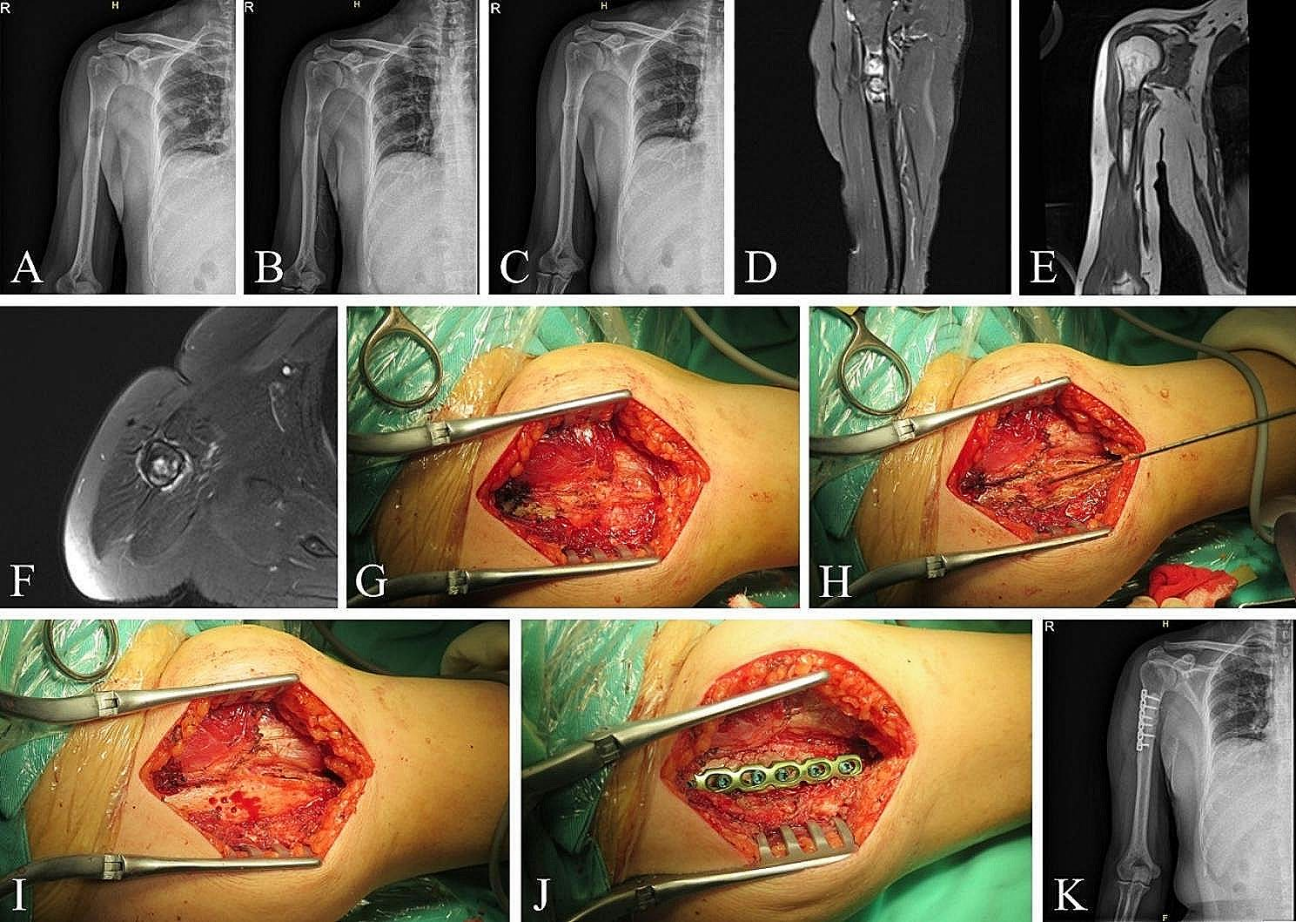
Right humeral pathological fracture in the upper segment. X-rays taken 5 months (A), 4 months (B), and 1 week (C) preoperatively show local thinning of the bone cortex and discontinuity of part of the bone cortex. (D-F) MRI taken 3 months preoperatively shows a soft tissue mass, approximately 2.0 × 1.9 × 4.8 cm, with unclear boundaries. (G) Exposure of the fracture and reveals callus formation. (H) Radiofrequency ablation. (I) Bone drilling, opening of bone window, and removal of tumor from the marrow cavity. (J) Bone cement filling, fixation with plate and screws. (K) X-ray taken 3 days postoperatively
